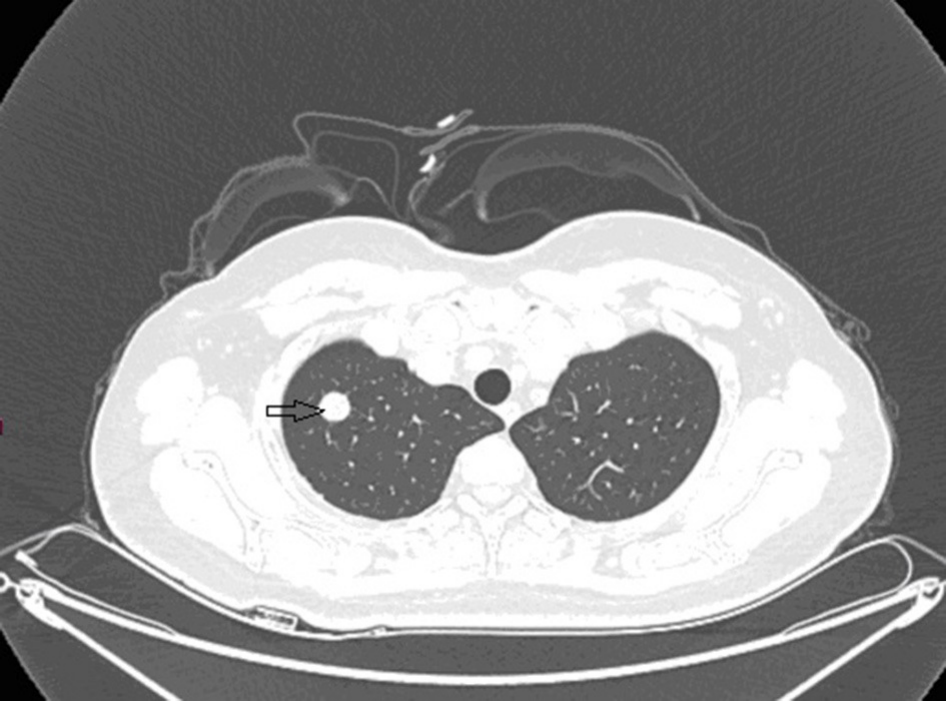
Contrast-enhanced CT images at 18 months after second surgery: lump in upper lobe of the right lung.
